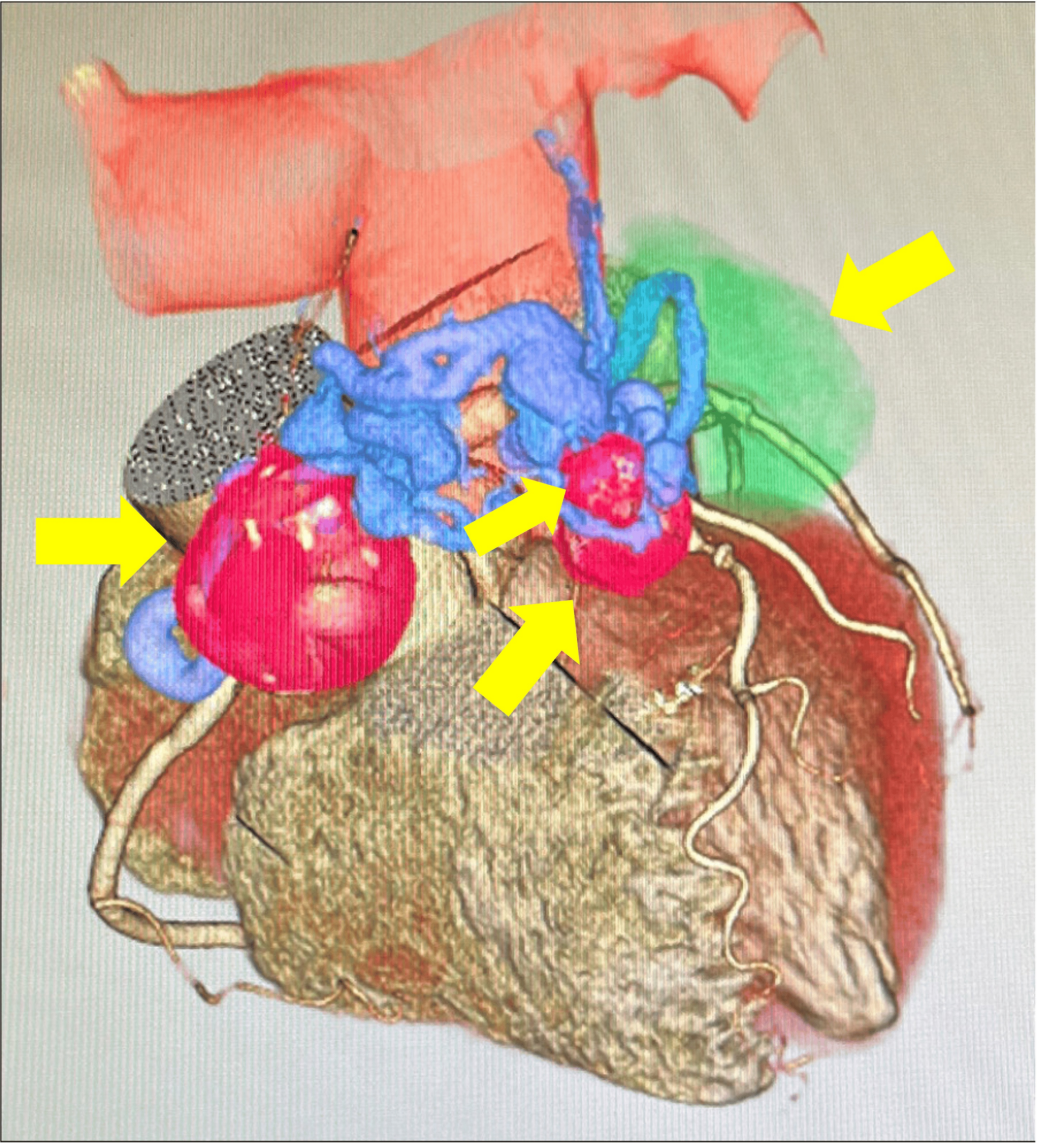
Preoperative coronary 3D CT image Yellow arrows indicate aneurysms. The largest aneurysm, colored green, has an aneurysm diameter of 45 mm. CT, computed tomography
